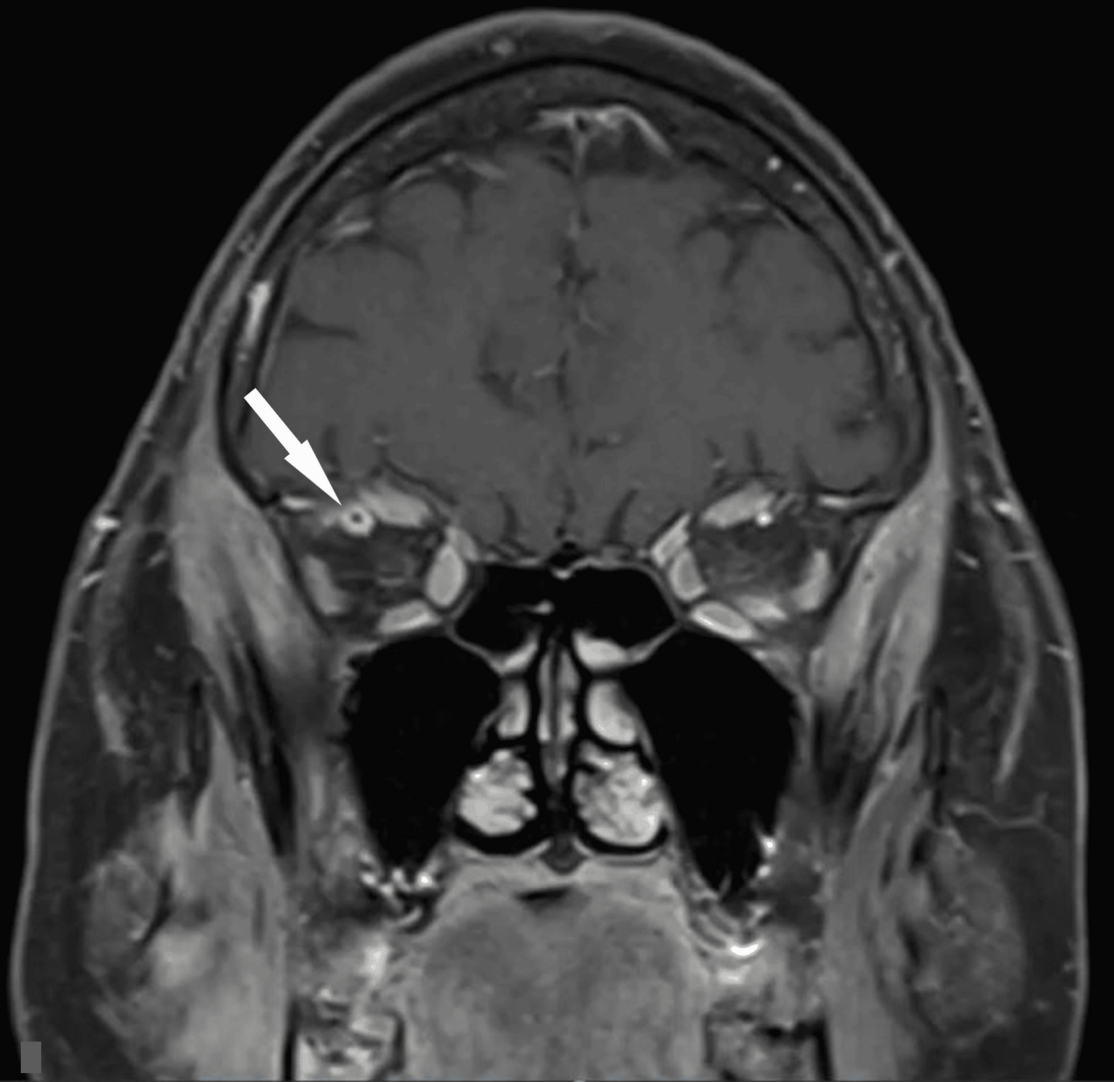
MRI images of the brain showing findings of persistent right superior ophthalmic vein thrombosis. The coronal T1-weighted post-gadolinium image shows a persistent intraluminal filling defect within the right superior ophthalmic vein compatible with persistent right superior ophthalmic vein thrombosis (white arrow, A).
